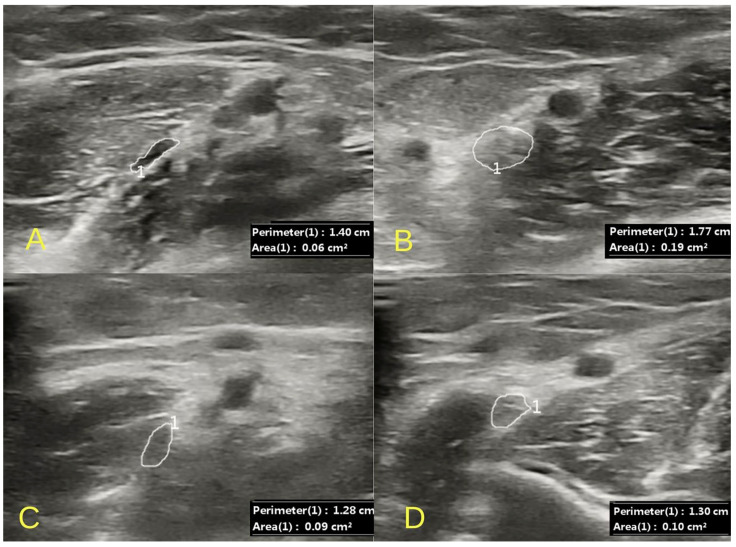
An ultrasound image of the massive compression of the median nerve (1) between the heads of the pronator teres muscle (A) with the swelling of the nerve at the level of the lacertus fibrosus (B). Postoperative evaluation reveals a normal median nerve both at the site of the previously noted compression (C) and in the ulnar fossa (D). Images were obtained with the D7L40L linear probe of the Chison CBit4.
